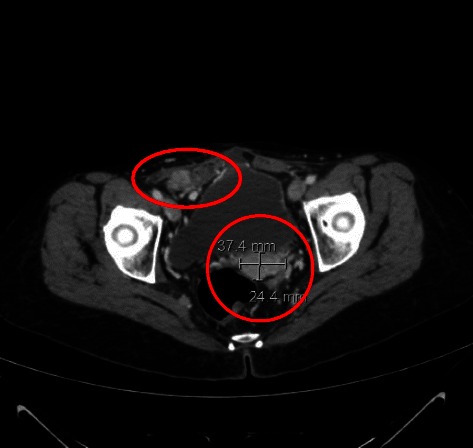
July 2012 (11 months after the start of treatment): CT scan showing a reduction in the size of the abdominal lesions
